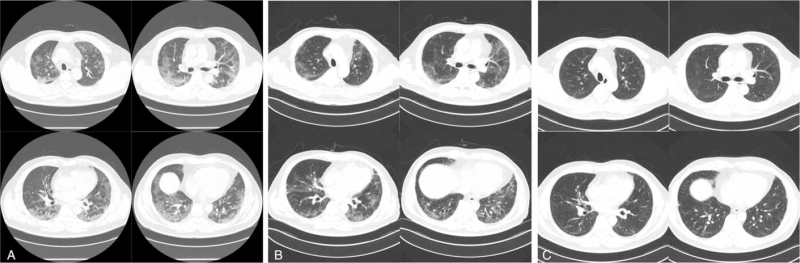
Chest CT images of the case. (A) The first computed tomography (CT) scan at admission day, ground-glass opacities (GGO) were observed in entire lung fields. (B) CT at 47th hospital day showed decreased GGO, but prominent fibrotic lung tissue change was suggested. (C) 3 months follow-up CT after discharge showed a decreased amount of lung fibrosis.
